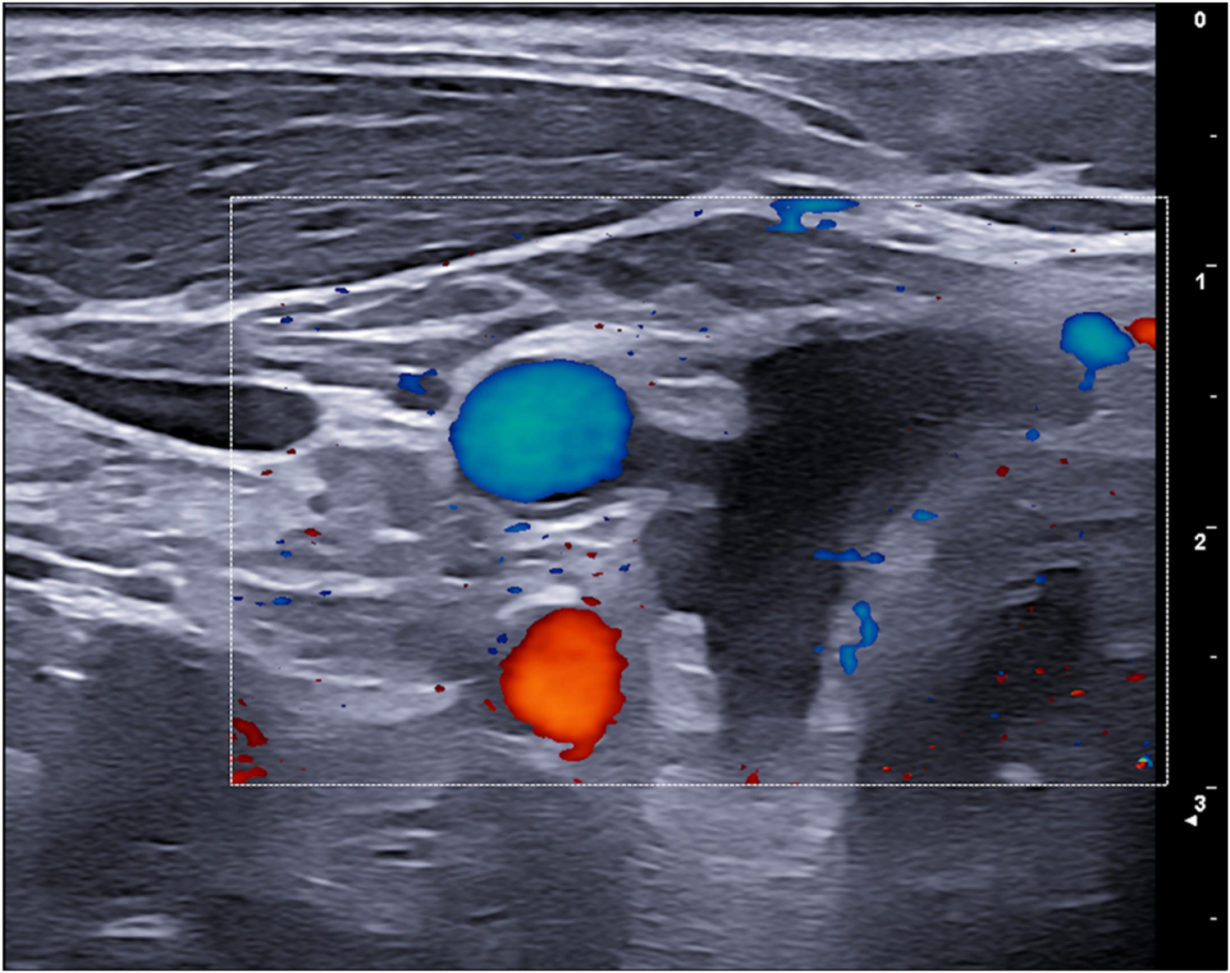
6-month ultrasound follow-up with no recurrence of cysts or cystic degeneration.
